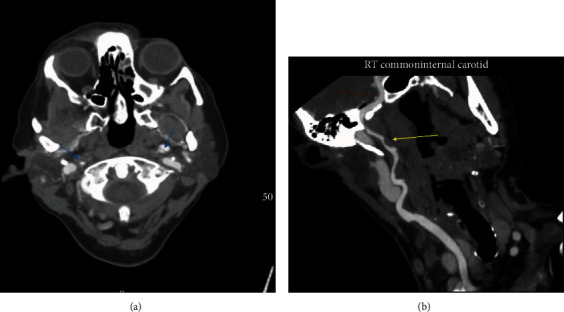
(a) Computed tomography angiography imaging showing the right and left common carotid artery dissection (blue arrows). (b) Computed tomography angiography imaging showing the right common (yellow arrow) and internal (red arrow) carotid artery dissections.
